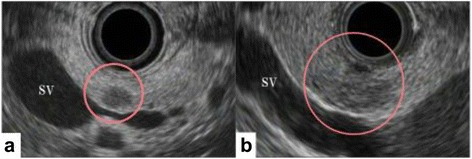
Endoscopic ultrasonography revealed indistinct and heterogeneous echogenic masses in the pancreatic body and tail. a body, b tail. *SV* splenic vein
